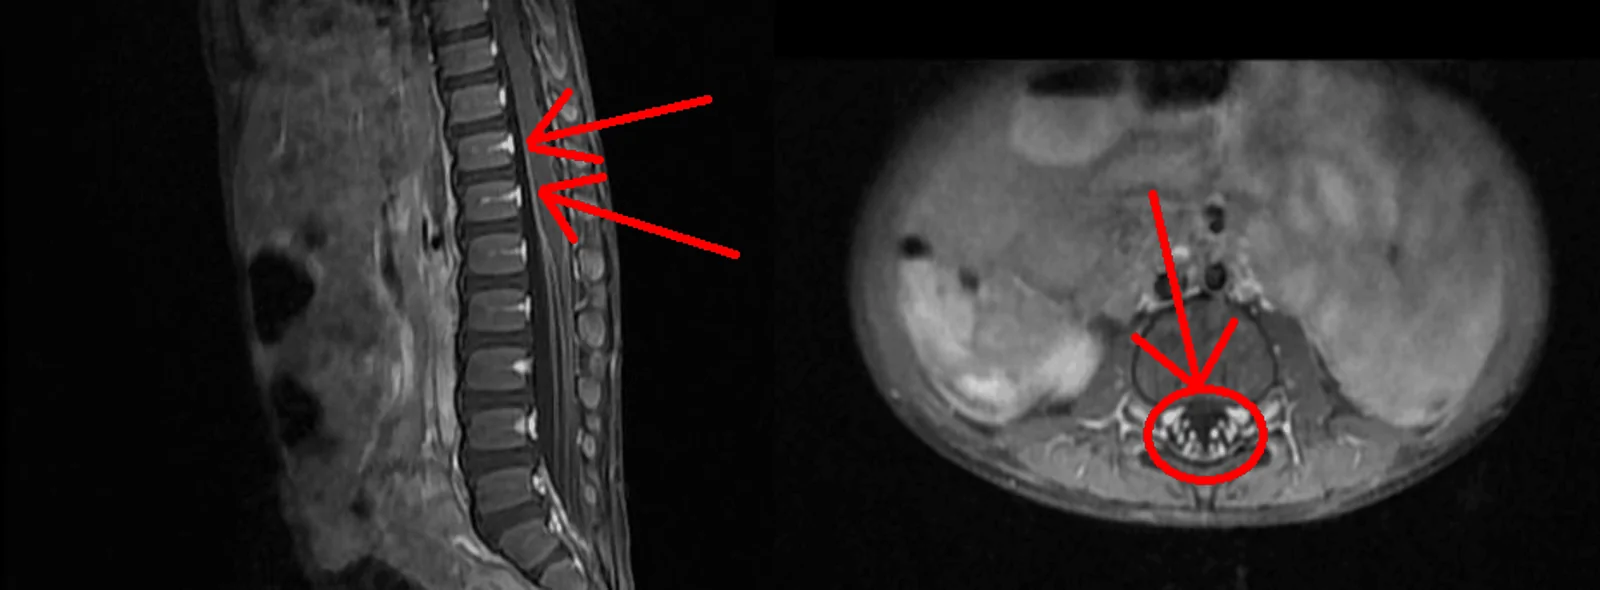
MRI findings of Guillain-Barré syndrome-like polyradiculoneuropathy Lumbar spine MRI demonstrates cauda equina nerve root enhancement/signal abnormality, supportive of inflammatory polyradiculoneuropathy in the appropriate clinical context. These findings highlight sequential central and peripheral nervous system involvement in neuroinvasive West Nile virus infection. Adapted from Zainab M [16]. Guillain-Barré syndrome. Radiopaedia.org case (rID: 211198), doi:10.53347/rID-211198, accessed June 1, 2026, with attribution in accordance with the applicable Radiopaedia Creative Commons license.
